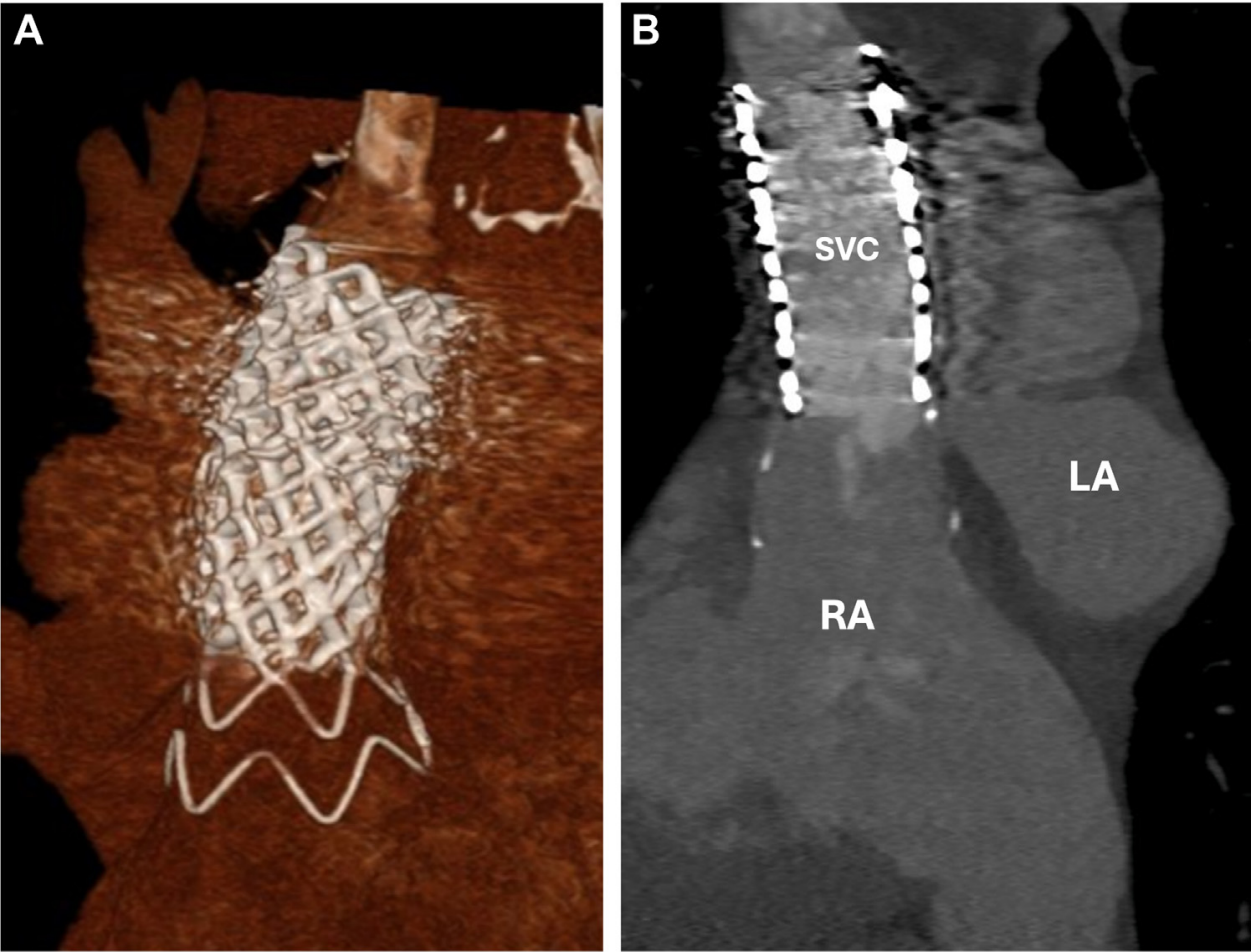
Follow-Up Computed Tomography Imaging (A) A 3-dimensional rendering from follow-up computed tomography of the Harmony Transcatheter Pulmonary Valve 22 mm (Medtronic) frame. The soft “inflow flare” of the valve expands to seal the sinus venosus atrial septal defect. (B) Reconstructed view of the valve frame completely sealing the sinus venosus atrial septal defect. RA = right atrium; other abbreviations as in Figure 4.
